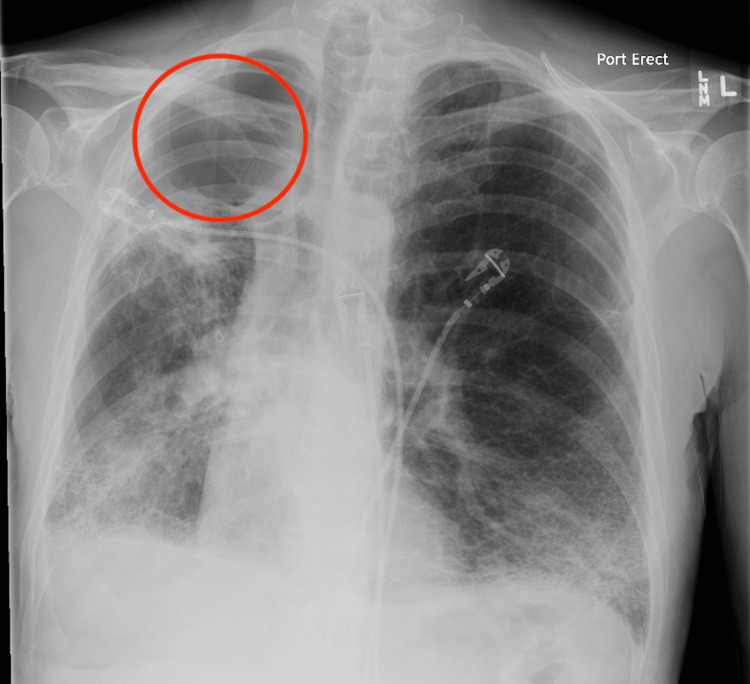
Anterior-posterior chest X-ray taken on admission. Bilateral patchy airspace opacities, right greater than left. Superimposed chronic fibrotic lung changes. Increased lung lucency, likely from emphysema. Circled in red is a large bulla at the right upper lobe.
